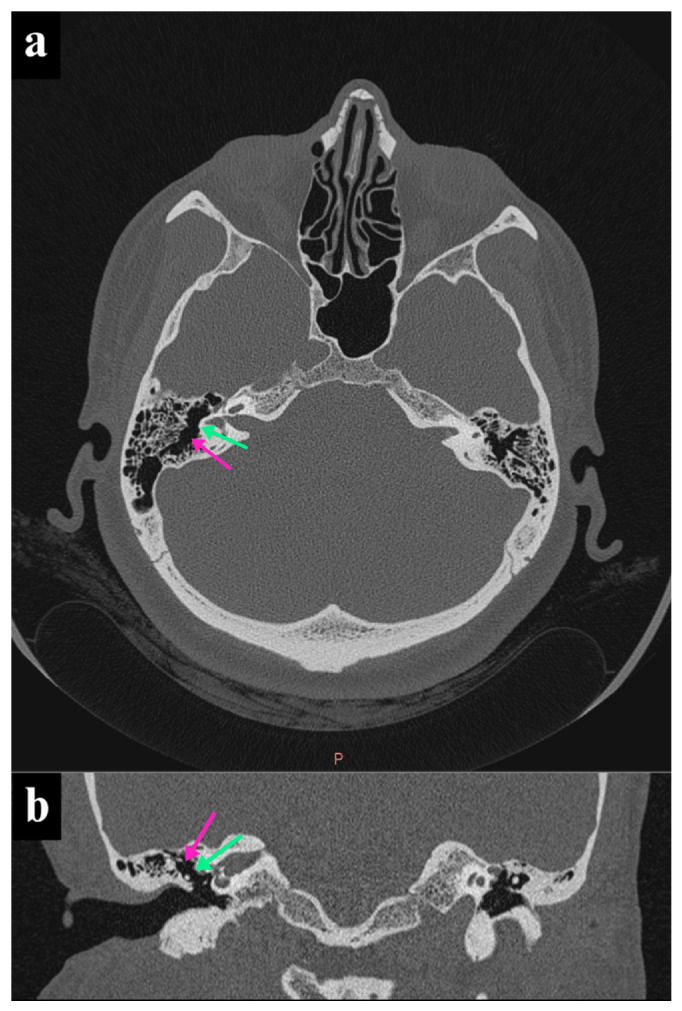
High-resolution CT of the right temporal bone showing a patent aditus ad antrum. (a) The axial image shows a patent aditus ad antrum (green arrow) and a normal, non-opacified antrum (purple arrow). (b) The coronal image again shows a patent aditus ad antrum (green arrow) and a normal, non-opacified antrum (purple arrow).
